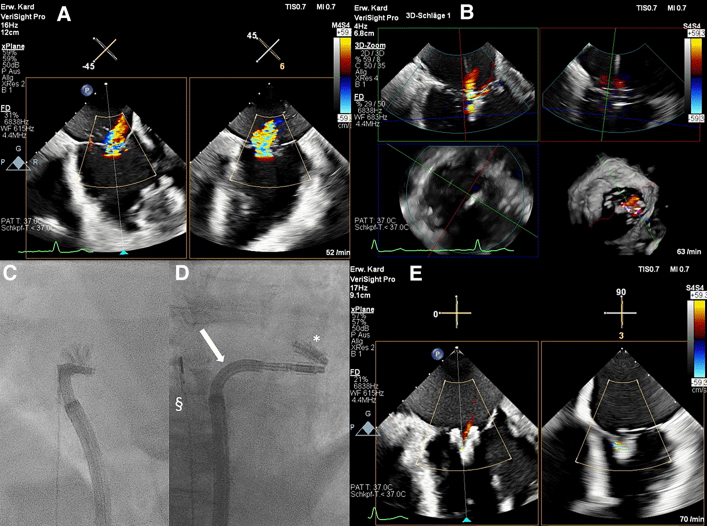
Procedural images by intracardiac echocardiography and conventional fluoroscopy. Legend: Panel *A*): preprocedural biplane imaging with colour doppler showing severe tricuspid regurgitation; Panel *B*): periprocedural multiplanar reconstruction of residual tricuspid regurgitation after implantation of the first device; Panel *C*) and *D*): fluoroscopic imaging describing the anatomical relationship between both devices in en-face [Panel *C*)] and right anterior oblique view [Panel *D*)], *: tricuspid transcatheter edge-to-edge repair device, arrow: device delivery system, §: three-dimensional intracardiac echocardiography probe; Panel *E*): biplane imaging with colour doppler demonstrating the final result with mild residual tricuspid regurgitation after implantation of two devices.
